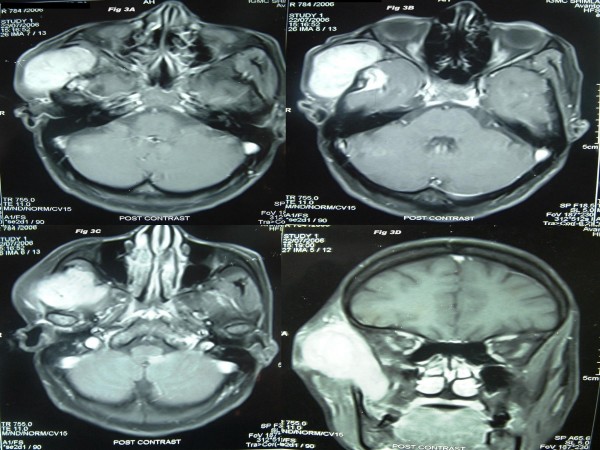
A-D: The mass is showing significant enhancement in post-contrast MR images (axial and coronal sections).
